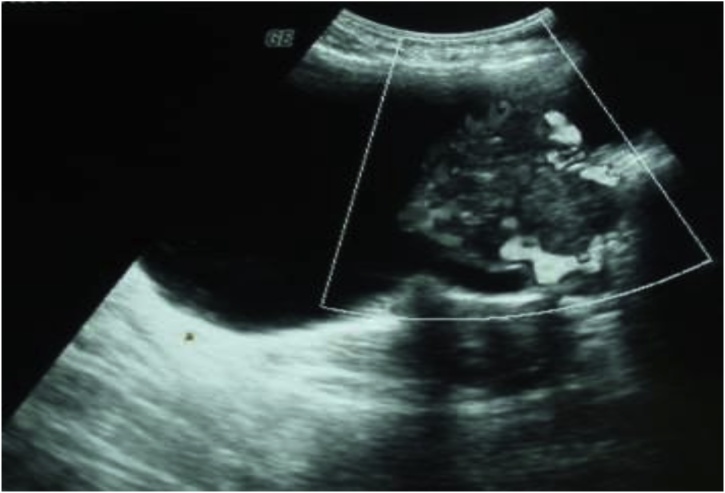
Ultrasound appearance of the bladder mass.
